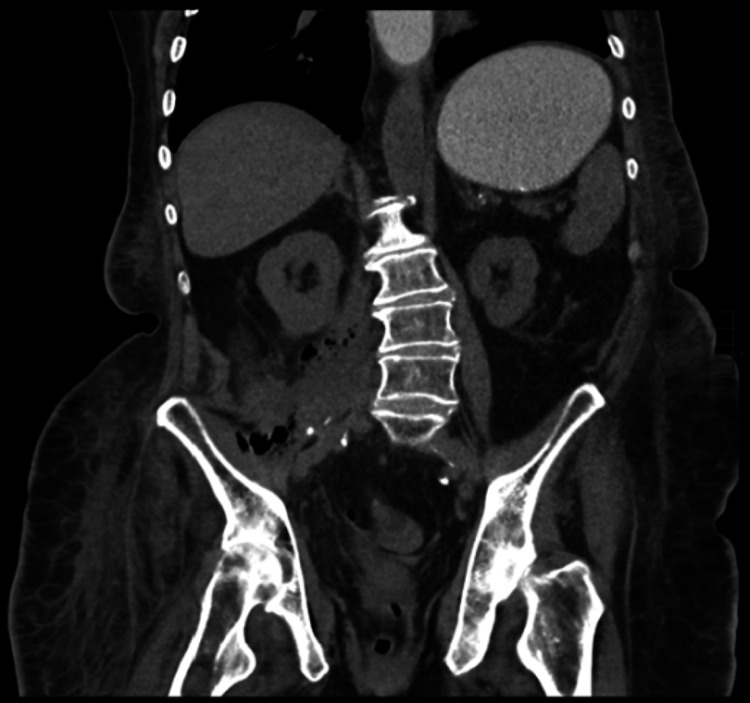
CT of the abdomen without contrast showing air, fluid, and abnormal thickening of the right iliopsoas musculature extending down to the right thigh, concerning for abscess.
